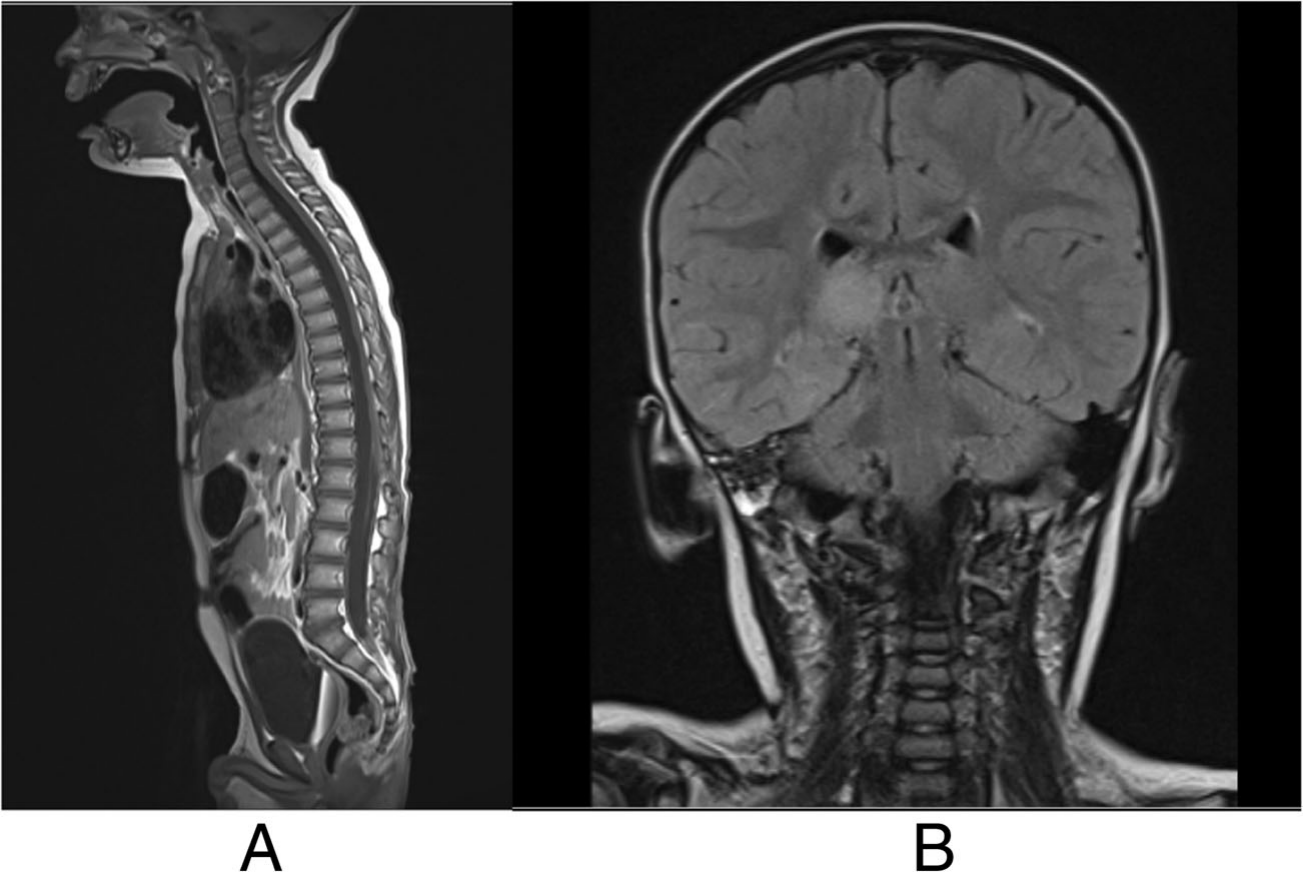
a T1-weighted MRI showing diffuse enhancement over the spinal cord again in keeping with CNS melanosis. b Coronal MRI flare image showing decompressed ventricles with oedema over the thalamus
